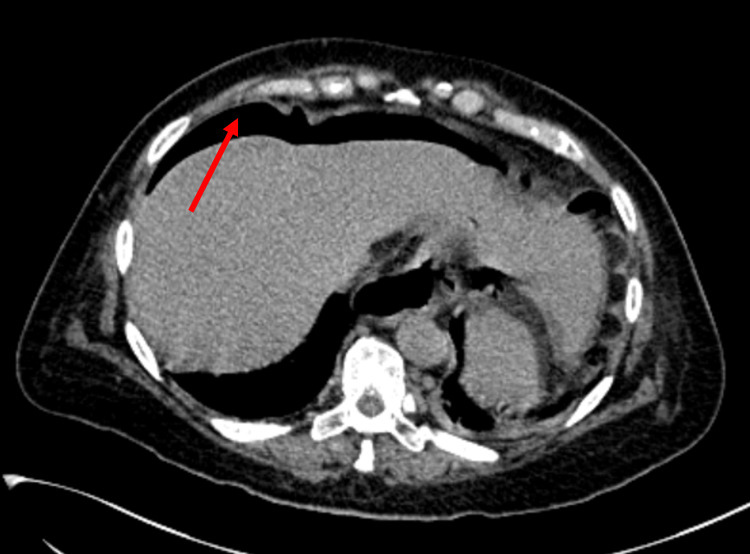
Computed tomography of the abdomen demonstrating free intraperitoneal air (arrow), consistent with pneumoperitoneum.
